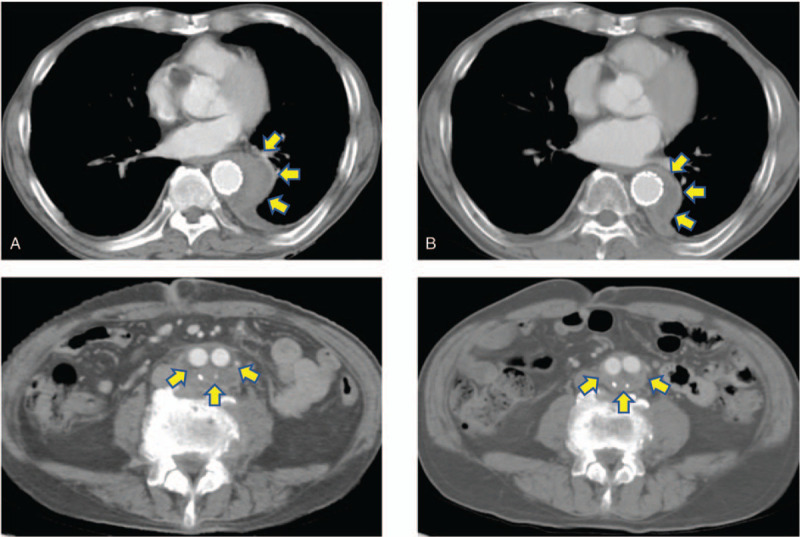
Postoperative CT. (a) 1 week postoperatively, (b) 6 months postoperatively. Postoperative CT showed no endoleak, migration, or anastomotic abnormalities, and no signs of recurrent infection were observed (arrow). CT = computed tomography.
